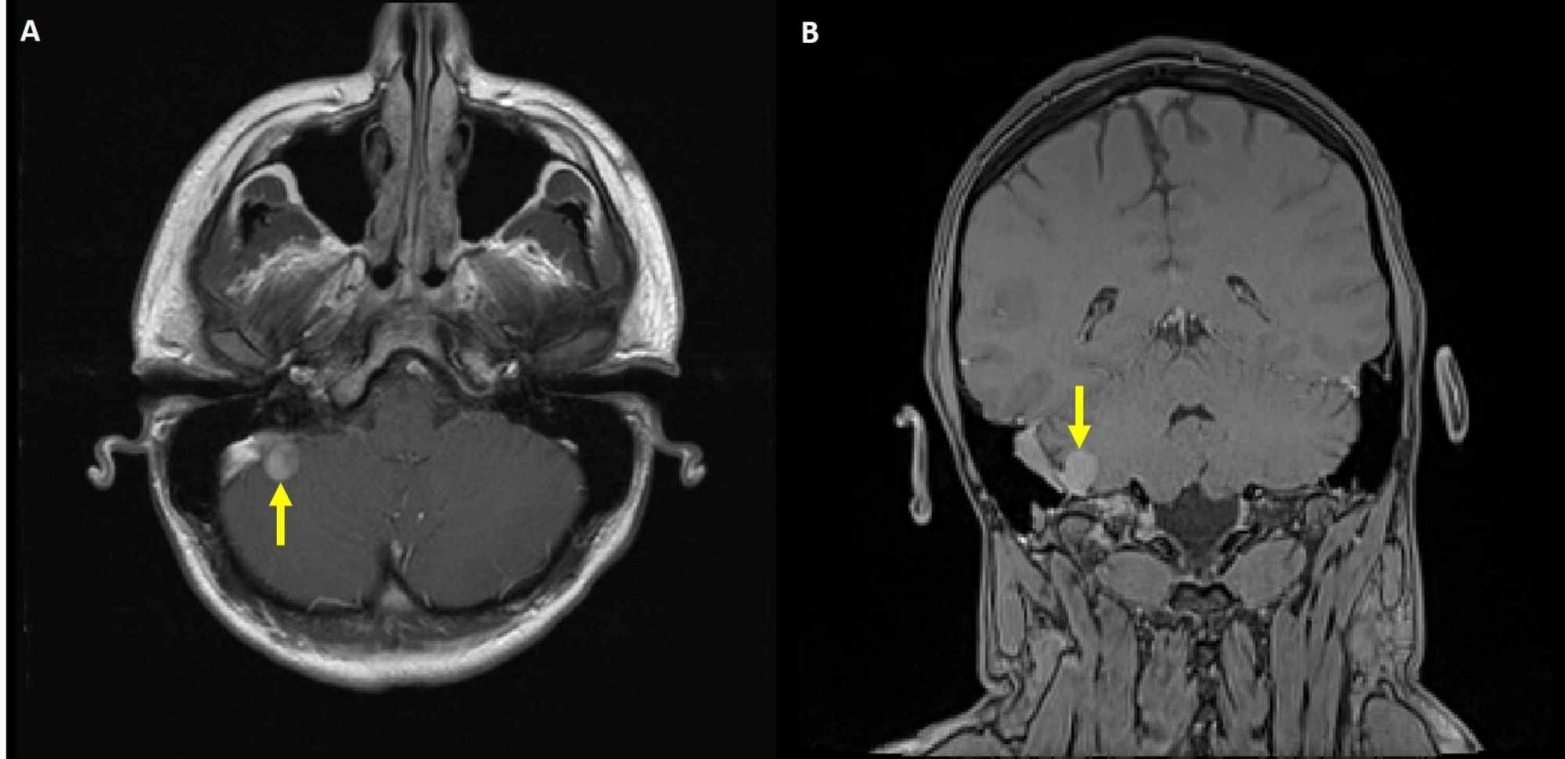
Preoperative magnetic resonance imaging (MRI) brain with gadolinium contrast (A) Axial and (B) coronal views showing an enhancing 1.5 cm extra-axial mass (solid yellow arrows) causing stenosis of the right sigmoid sinus.
